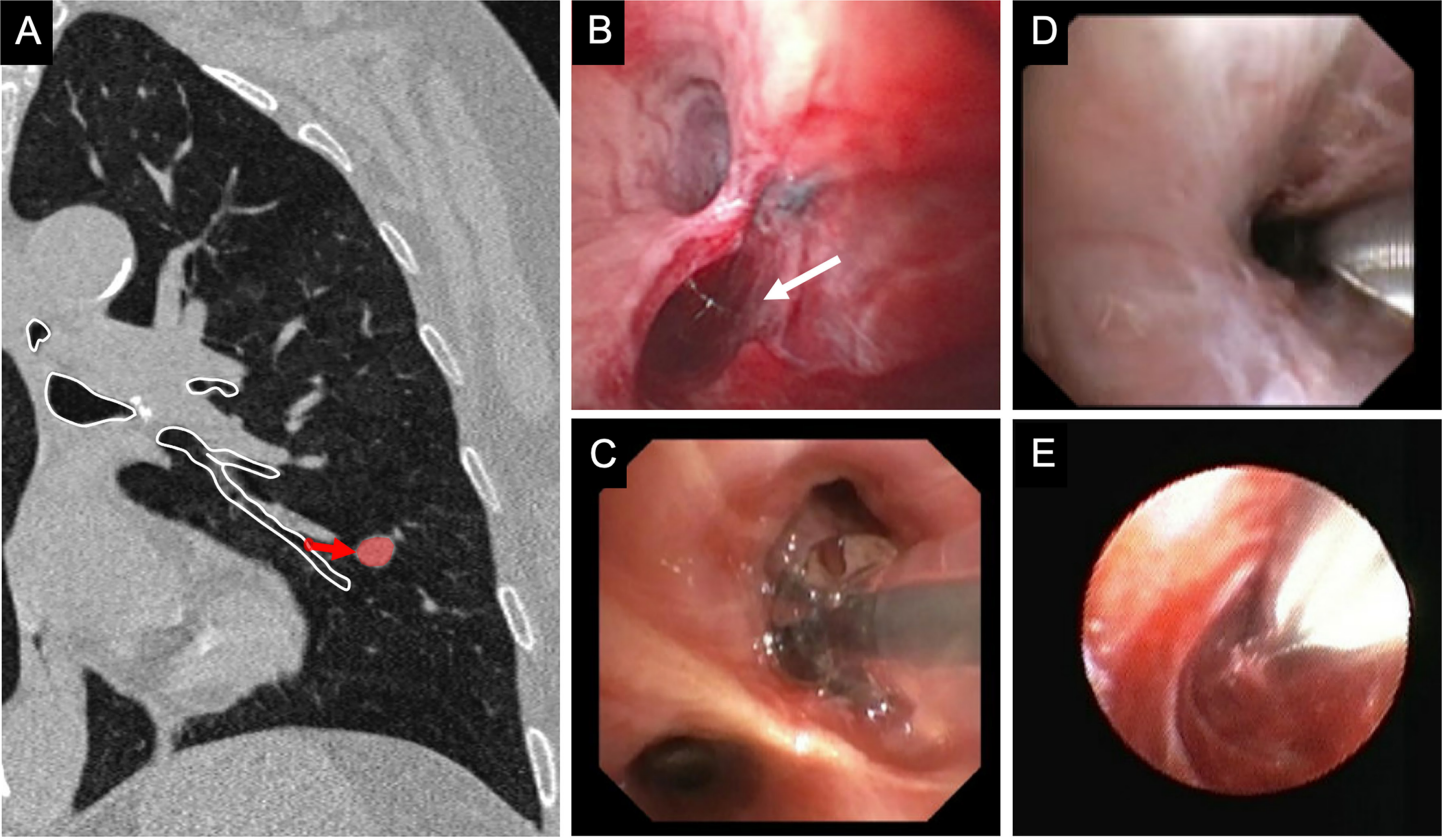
(A) Intraparenchamally located lesion (highlighted in orange) in the lingula, as seen in the coronal CT view. White lines indicate the airways, and the red arrow represents the transparenchymal route from the Point of Entry, measuring 11 mm. Endoscopic views: (B) Entrance of the Essen Tunnel, (C) balloon dilation, (D) insertion of the R‐EBUS probe into the tunnel and (E) perspective of an ultrathin bronchoscope on the target lesion within the Essen‐Tunnel prior to sampling.
